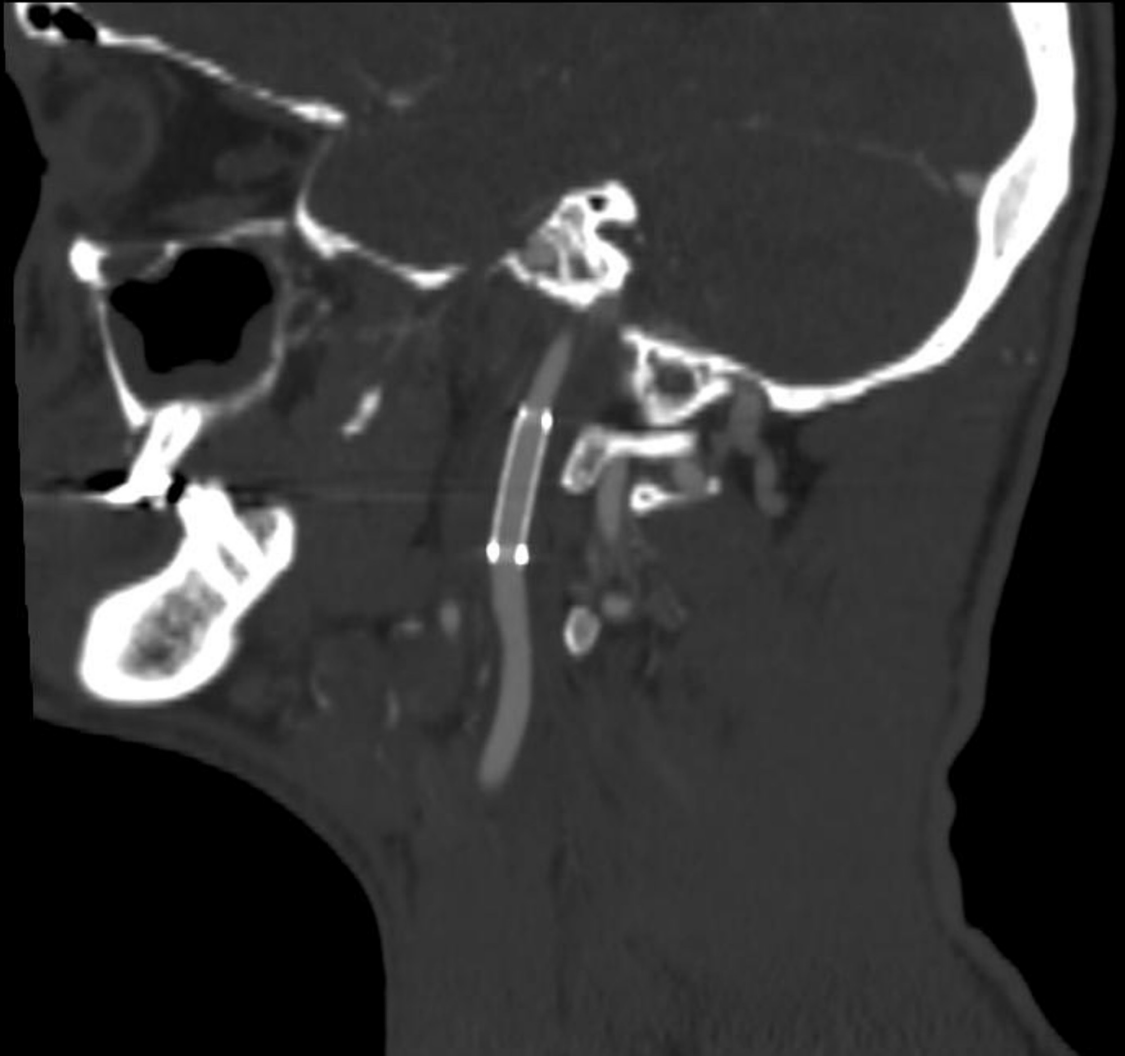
Computed tomography angiography neck 24 hours after endovascular repair showed patent stent and no evidence of pseudoaneurysm formation
